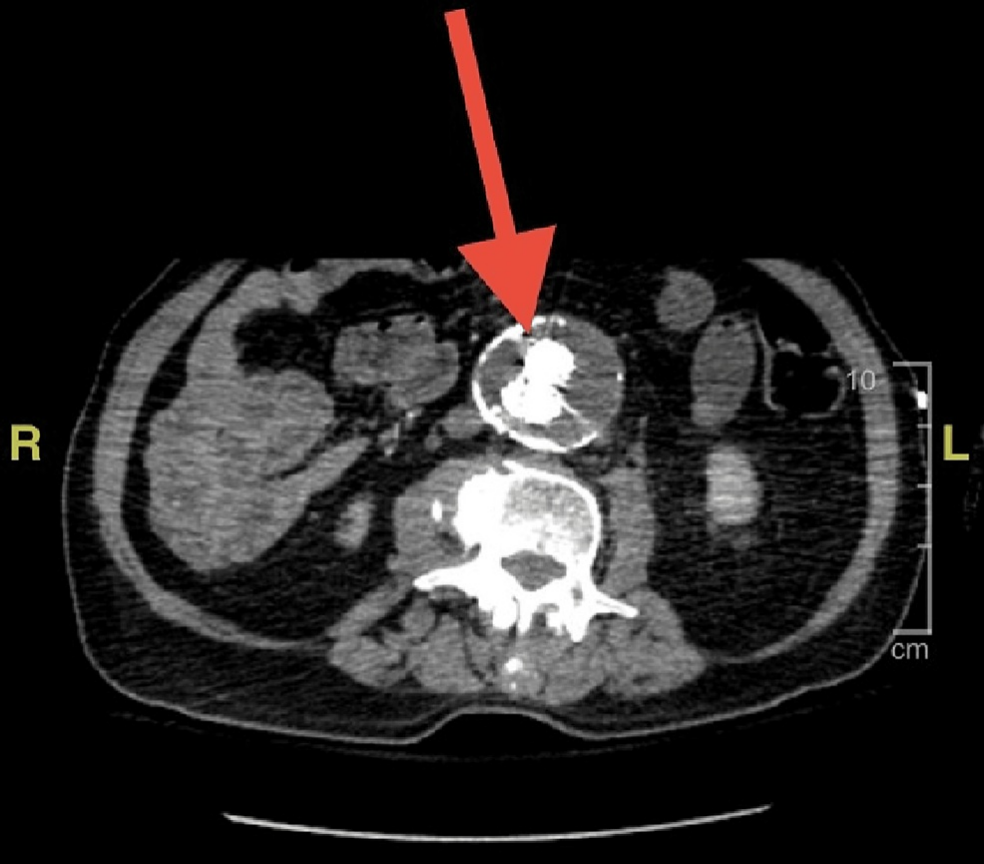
CT of the abdomen and pelvis with contrast on admission showing infrarenal abdominal aortic aneurysm with the aortobiiliac stent graft with the aneurysm sac measuring up to 4.6 x 5.5 cm in greatest dimension (red arrow). This size was not significantly changed when compared to imaging done in 2019.
